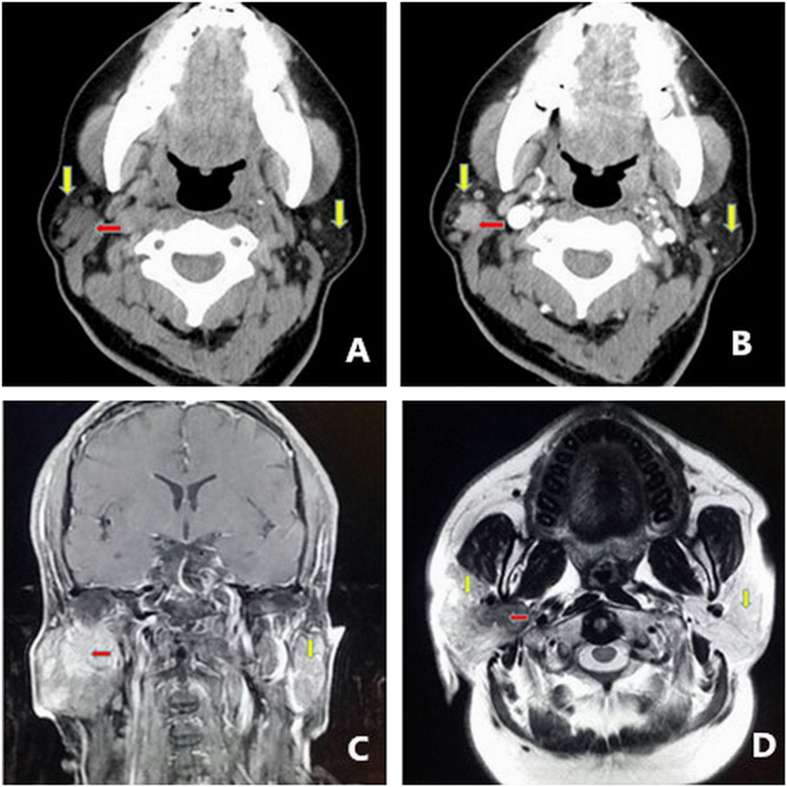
Major imaging findings for patients. (A) CT scanning in case 1 displayed circular nodule shadow in the right parotid gland. (B) Enhanced scanning identified cystic or necrotic tumor. (C) MRI displayed irregular shaped lesions in the right parotid gland globe in Case #5. (D) MRI enhanced scan revealed MECs tumors (yellow arrow highlighted parotid gland, red arrow highlighted the tumor).
